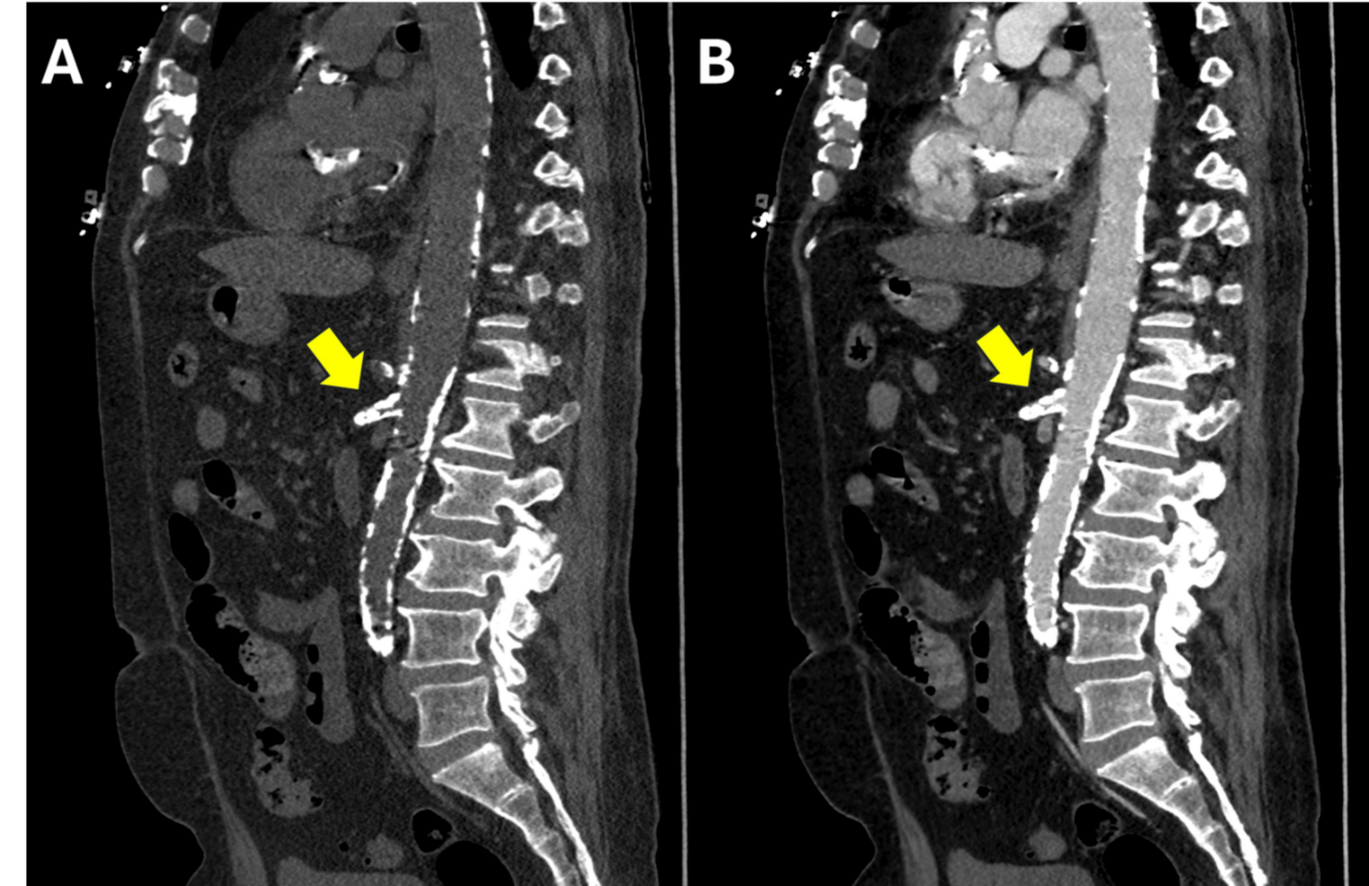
Sagittal (A) pre- and (B) post-contrast CT angiogram of the abdomen and pelvis demonstrating extensive calcification of the descending aorta and superior mesenteric artery (arrows).
